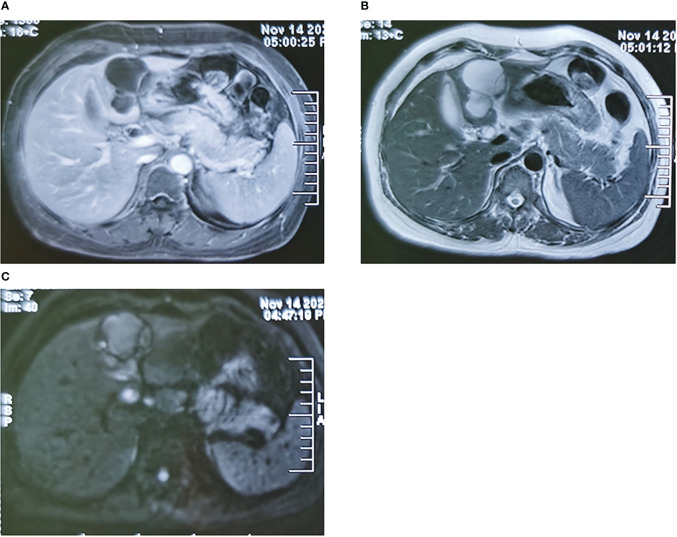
Typical images of magnetic resonance imaging of the liver lesions. (A) The arterial phase image of the liver lesions. (B) The T2 phase image of the liver lesions. (C) The DWI phase image of the liver lesions. DWI, diffusion-weighted imaging.
